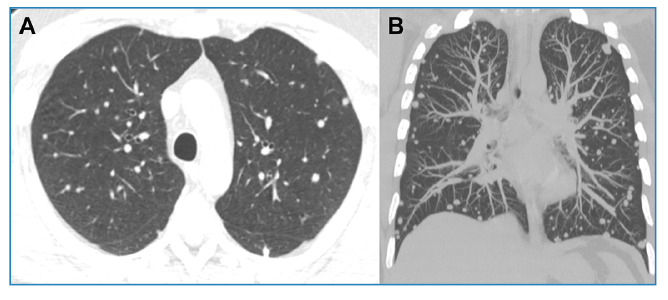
Chest computed tomography images with axial reconstruction (A) and coronal maximum-intensity projection reconstruction (B) obtained 10 months later, demonstrating a significant reduction in nodule size.
